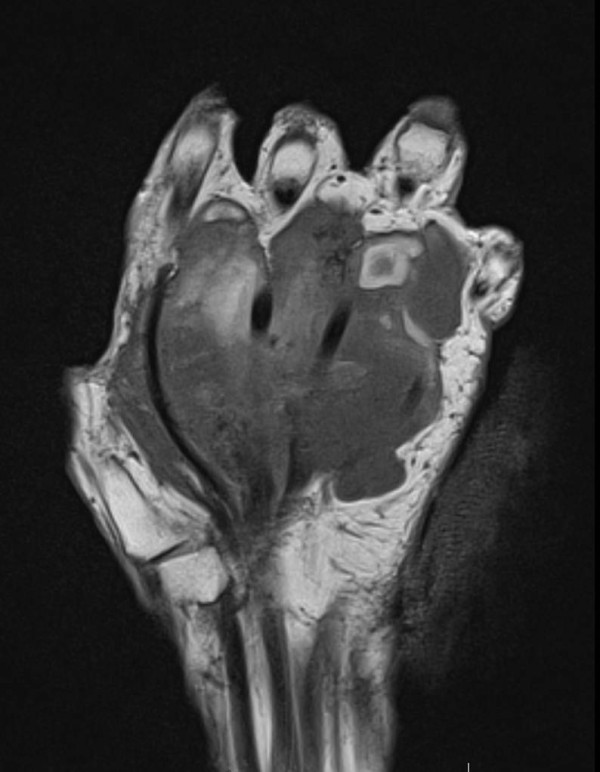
Magnetic resonance image of a coronal section of the hand showing a large mass around the palmar structures of the hand, but with no bone involvement.
